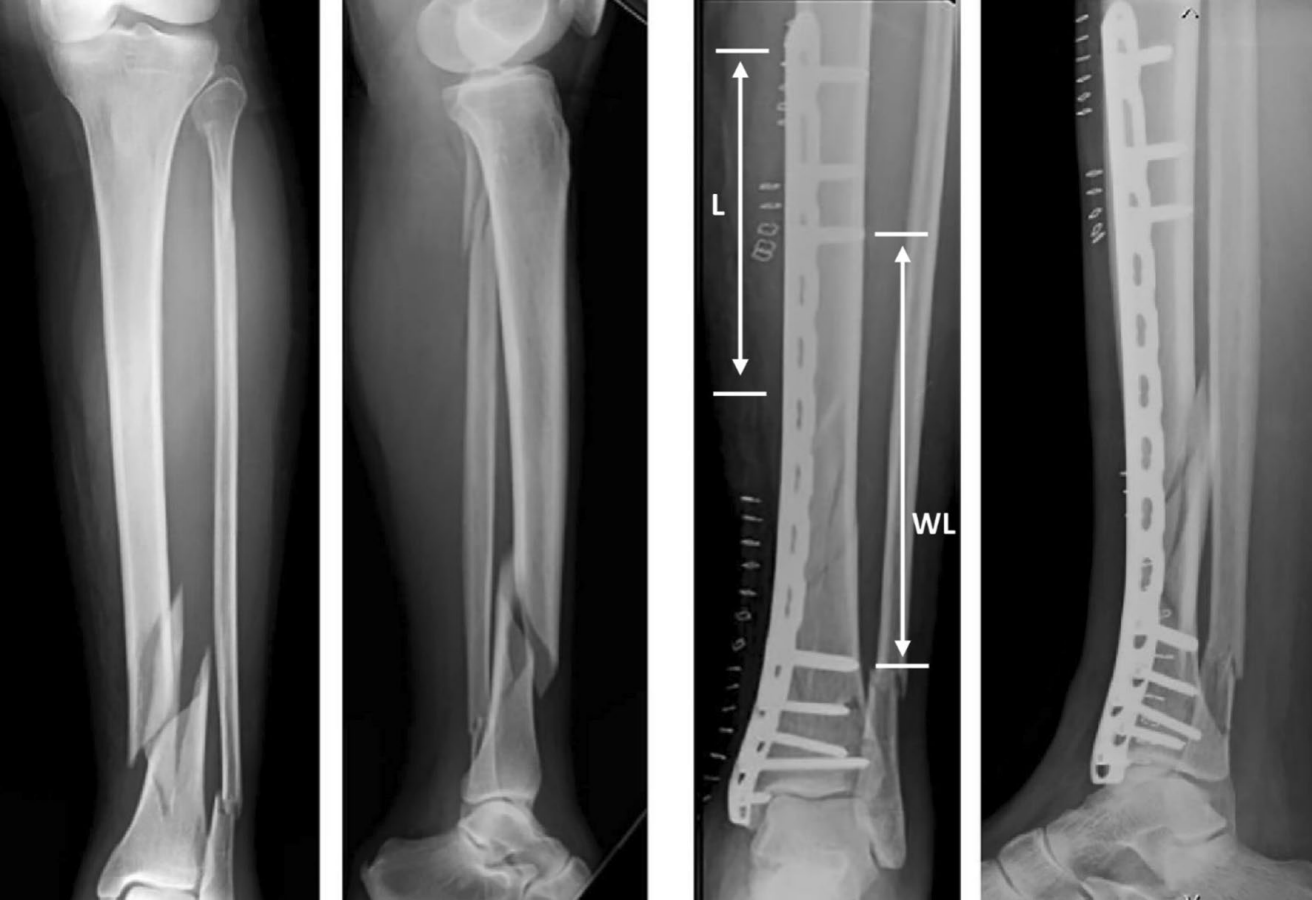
Pre operative radiographs of a left tibia show a 42-B2 fracture with an associated transverse fibula fracture. The tibial fracture was treated with a medial distal tibial bridge plate. Working length is depicted on post operative radiograph (*WL*). Screw density is defined as the length of the plate proximally to the fracture site (*L*) divided by the number of screws proximally. In this radiograph *L* is 112 mm, resulting in a screw density of 37.3 mm
